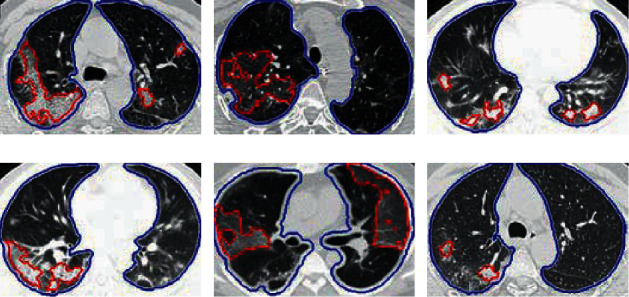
Qualitative identification results of abnormal CT results with COVID-19, including pulmonary parenchyma (blue outline) and GGO and PIs (red outline).
